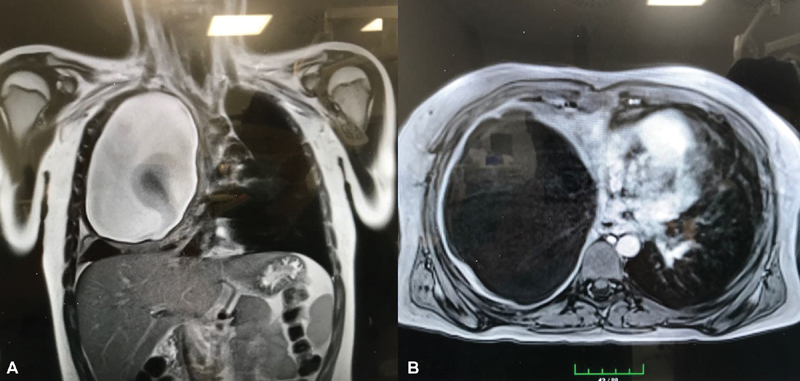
Magnetic resonance images of cystic lesion located in the right hemithorax, (
